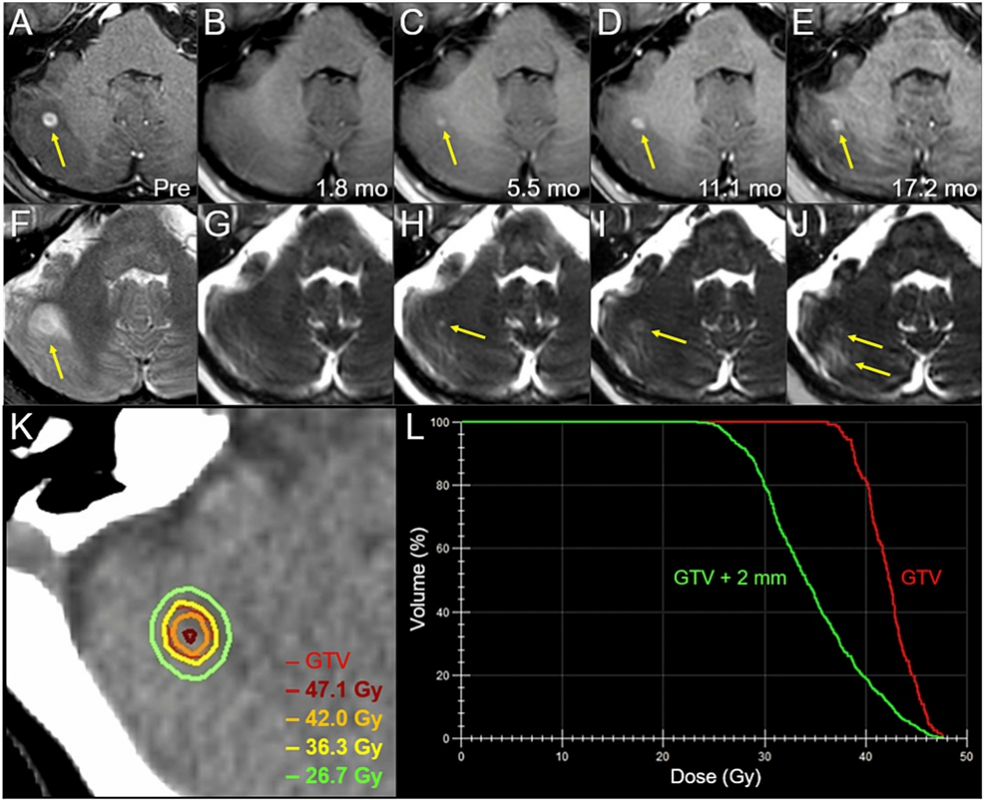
Magnetic resonance images, dose distribution, and dose-volume histograms before and after the first three-fraction stereotactic radiosurgery in the right cerebellar lesion. The images show (A-E) axial CE-T1-WIs and (F-J) axial T2-WIs (A, F) five days before (Pre) the initiation of SRS, (B, G) at 1.8 mos after SRS initiation, (C, H) at 5.5 mos, (D, I) at 11.1 mos, and (E, J) at 17.2 mos; (K) an axial dose distribution; and (L) DVHs. (A-J) These images are shown at the same magnification and coordinates under co-registration and fusions. (A, F) A solid enhancing lesion (arrow in A) with the corresponding mass on T2-WI (T1/T2 match) associated with perilesional edema (arrow in F) in the right cerebellar hemisphere. (B, G) At 1.8 mos, the lesion regressed completely. (C-E, H-J) The enhancing effect gradually increased (arrows in C-E); however, the corresponding mass on T2-WI remained obscure (T1/T2 mismatch) and the perilesional edema was mild (arrows in H-J). (K) The GTV contour (thin line) and representative IDLs (thick lines) are superimposed onto an axial CT image. (L) The GTV + 2 mm structure was generated by adding an isotropic 2-mm margin to the GTV for the evaluation of the dose gradient outside the GTV boundary. CE, contrast-enhanced; WIs, weighted images; SRS, stereotactic radiosurgery; CT, computed tomography; DVH, dose-volume histogram; mo, month; GTV, gross tumor volume; IDL, isodose line
